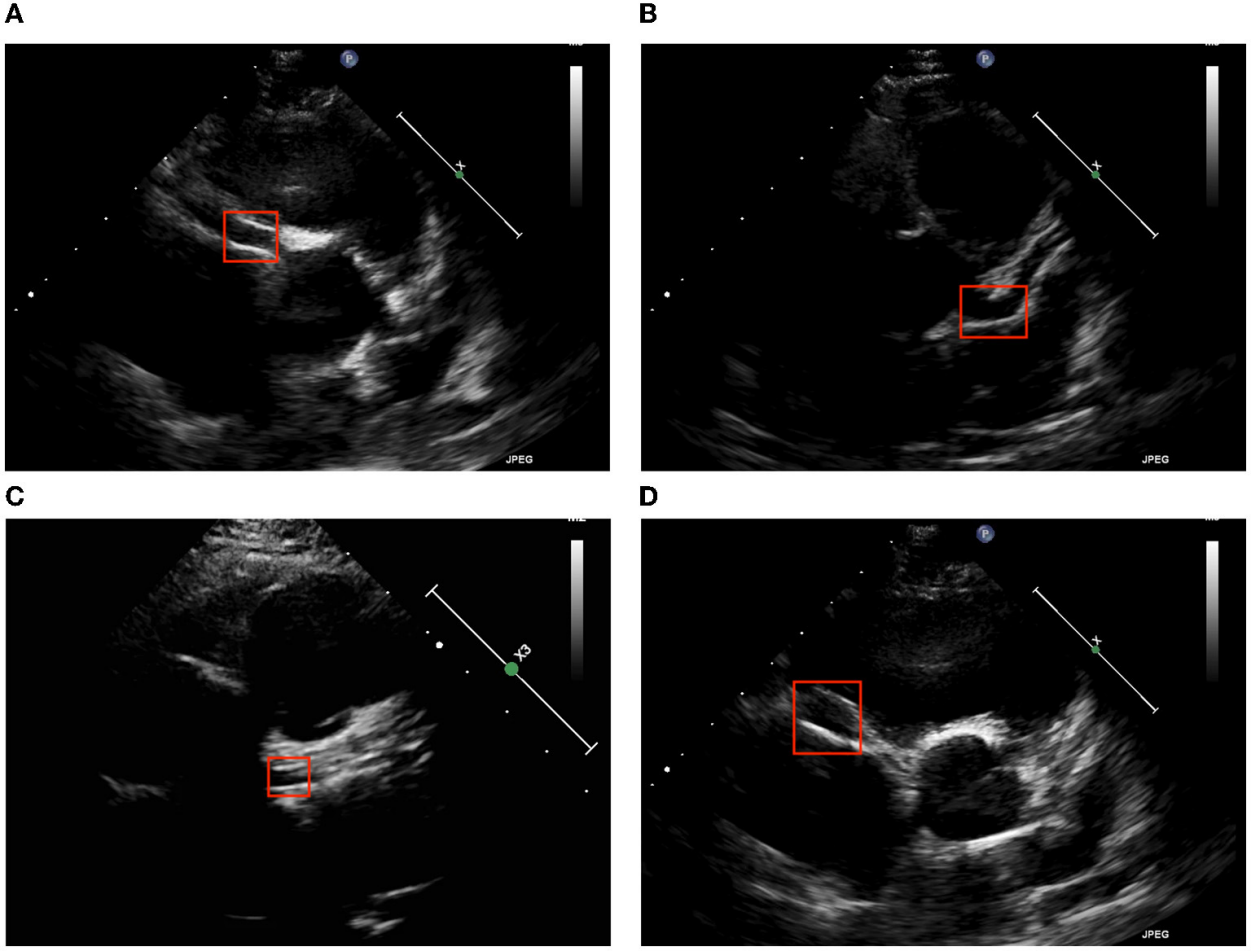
Two major echocardiographic coronary patterns in children with KD. (A) LCA brightness, (B) LCA dilatation, (C) RCA brightness, and (D) RCA dilatation.
